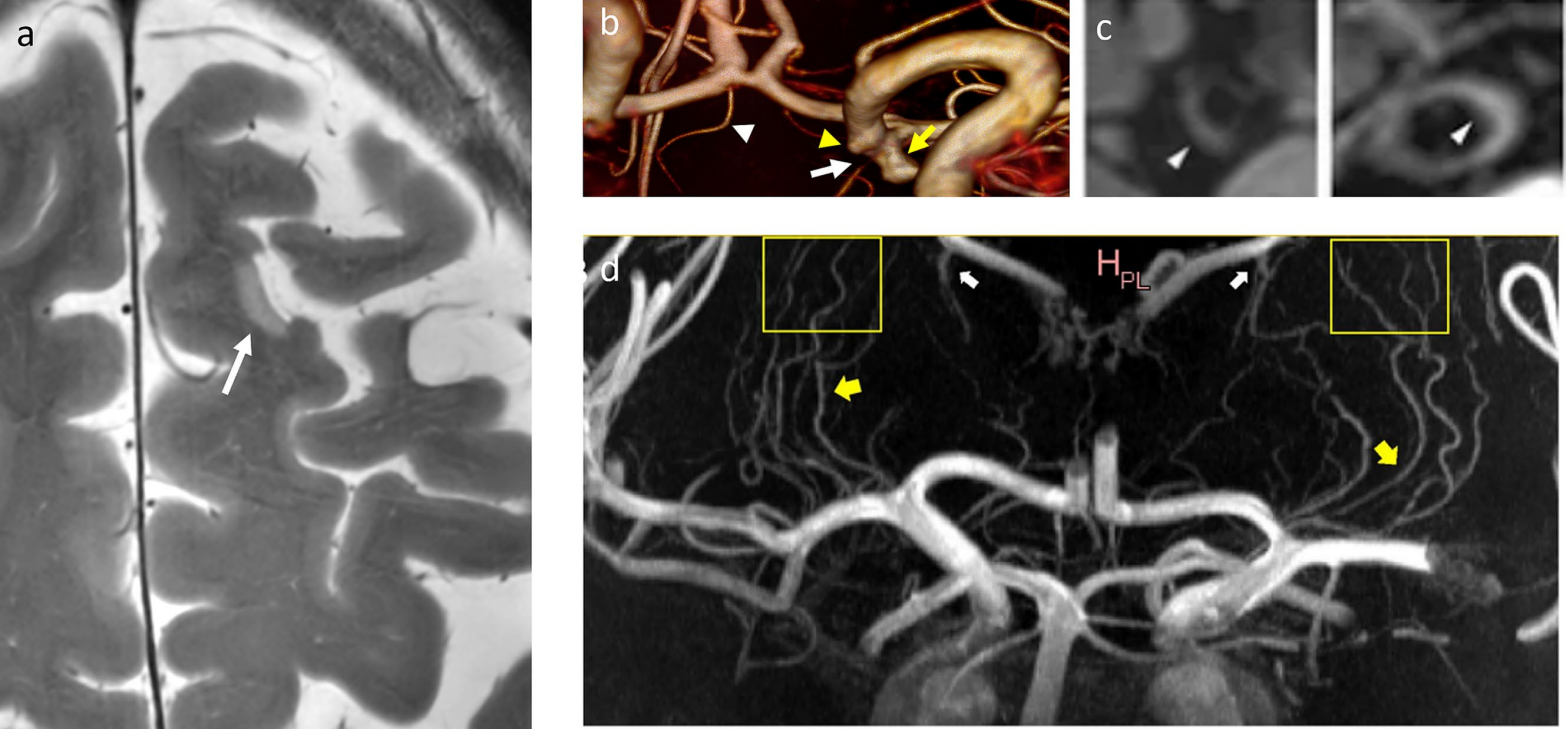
Microinfarcts, lenticulostriate arteries, and vessel wall lesions. (a) T2w-hyperintense cortical microinfarction in the left superior frontal gyrus, in-plane-resolution: 0.2 mm x 0.2 mm. (b) 3D reconstruction of a 3D TOF MRA indicating the origin of the left choroidal artery (white arrow) from the neck of an aneurysm of the terminal carotid artery (yellow arrow). White arrowhead: right recurrent artery of Heubner, yellow arrowhead: infundibular origin of the left posterior communicating artery. (c) Vessel wall lesions and calcifications. From left to right: CT images of a thick calcification in the right ICA and two calcified spots in the left VA, and 7T MR images of an elongated vessel wall lesion in the right ICA and a thicker vessel wall lesion in the left VA, adapted from reference (Lucci et al., 2024) (CC BY 4.0 license). Both ICA and VA exhibit a significant presence of calcifications and vessel wall lesions as opposed to other intracranial arteries. (d) Maximum Intensity Projection of a contrast-enhanced TOF-MRA demonstrates lenticulostriate arteries (yellow arrows), that can be followed over long trajectories (yellow boxes), as well as prominent choroidal and thalamostriate veins (white arrows), adapted from reference (Bai et al., 2024) (CC BY 4.0 license). ICA: internal carotid artery, VA: vertebral artery, TOF-MRA: time-of-flight-magnetic resonance angiography.
